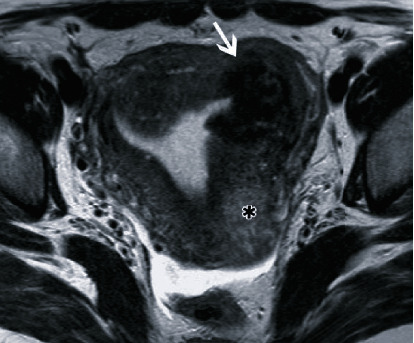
Magnetic resonance image 7 months following uterine artery embolization. T2-weighted axial image demonstrates a residual fibroid (white arrow) in the myometrium. The irregularity of the endometrium adjacent to the fibroid is shown. The asterisk (∗) shows another degenerative uterine fibroid.
